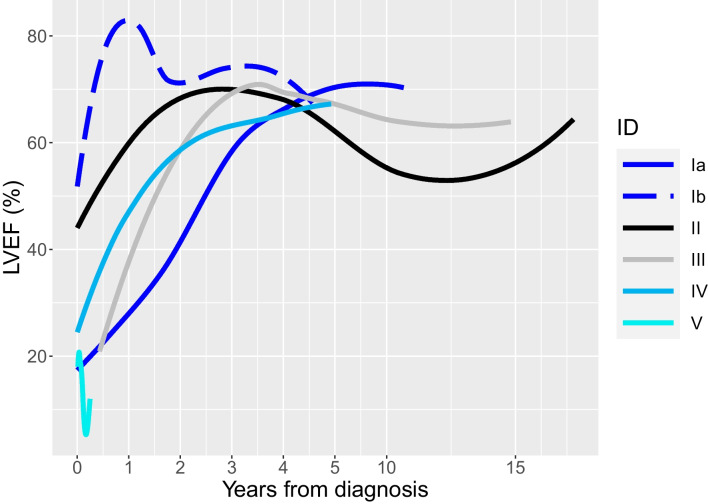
Left ventricular ejection fraction (LVEF) from diagnosis to last follow-up (*n*=84 echocardiograms from 6 patients). LVEF normalized in all patients except patient V who showed a progressive decline in LVEF
